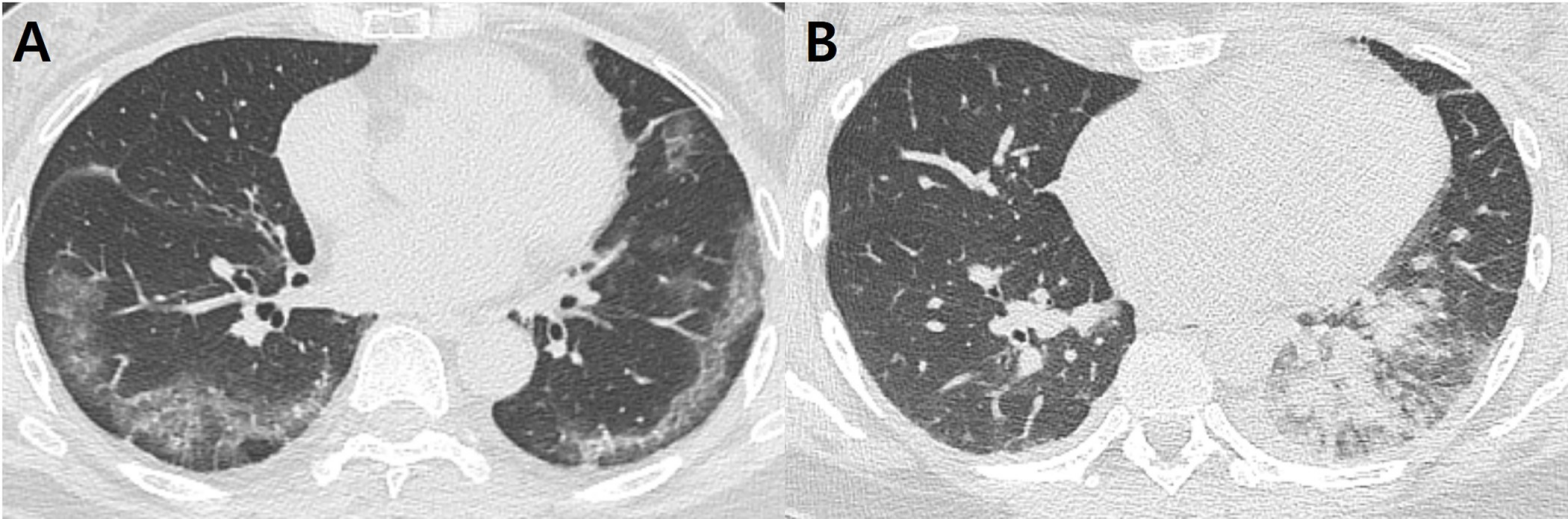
Representative cases of SARS-CoV-2 (A) and endemic HCoVs (B) pneumonia. (A) Pneumonia due to SARS-CoV-2 in a 63-year-old female. Axial computed tomography (CT) shows multifocal ground-glass opacities (GGOs), predominantly located in the peripheral areas of both lungs. (B) Pneumonia due to HCoV-229E in a 35-year-old female. Axial CT shows patchy and nodular consolidation and GGO in the left lower lobe. A small amount of pleural effusion is also present.
